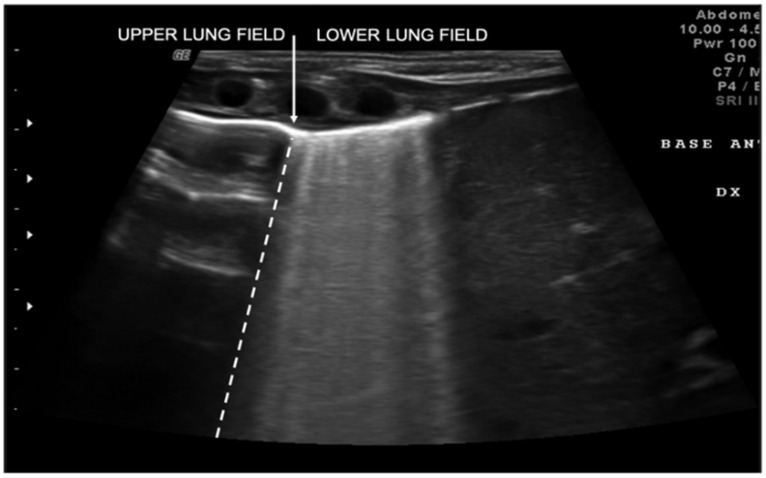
Transient tachypnea of the newborn. The dashed line shows the ultrasound demarcation line between the upper and lower lung fields: double-lung point (white arrow).
